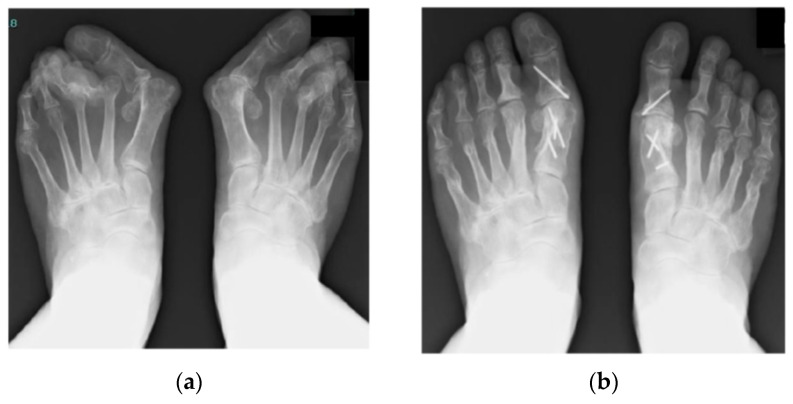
Representative case of a 75-year-old woman with a 40-year history of RA. (a) Preoperative radiograph with weight-bearing. Severe hallux valgus deformity, subluxation/dislocation of the lesser toe MTP joints, and joint erosion are seen. (b) Postoperative radiograph with weight-bearing 7 years after surgery. Combination surgery of modified scarf osteotomy and metatarsal shortening offset osteotomy has been performed. Akin osteotomy was also added to control the rotation and alignment. Painful callosity disappeared, and correction has been maintained 7 years after surgery. She can run at present.
